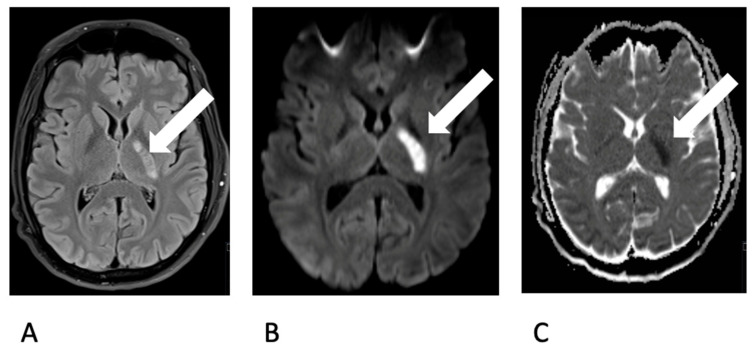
Depiction of an ischemic lesion (white arrow) in the posterior limb of the left internal capsule (A. choroidal artery territory) on MRI. Demarcation on T2 FLAIR-w image (A) and Diffusion restriction on b1000 image (B) and ADC map (C).
